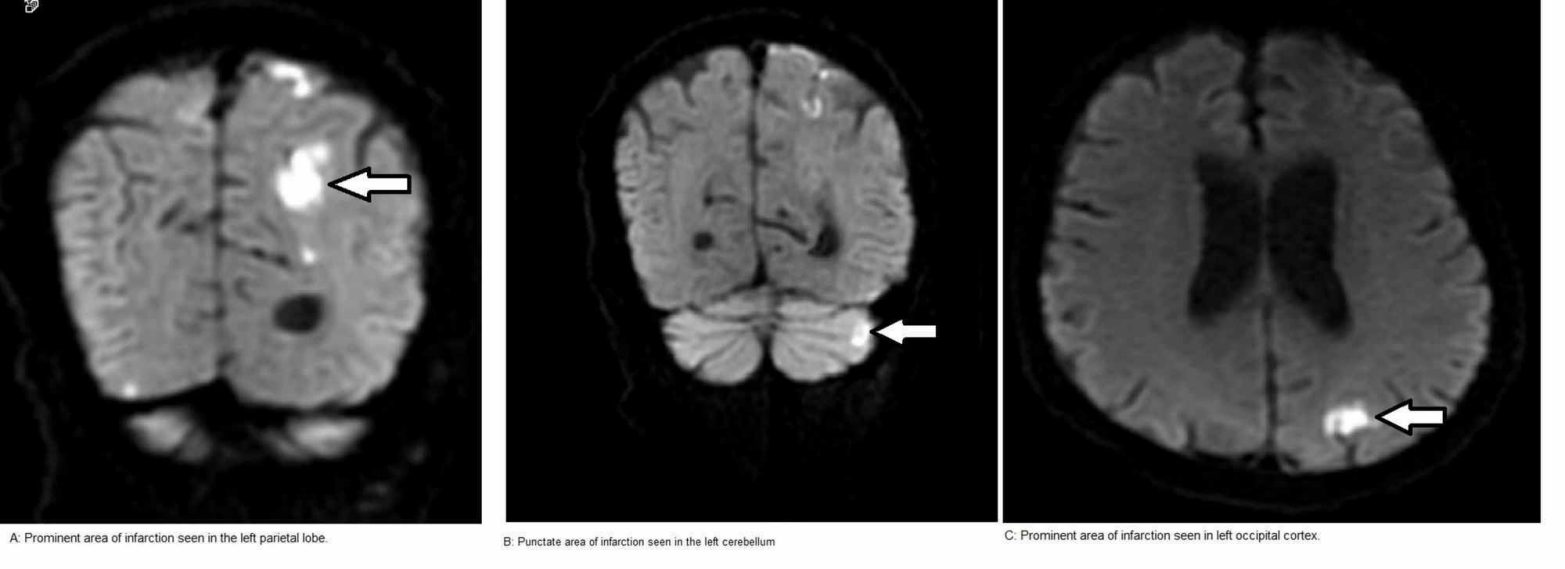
MRI of the head. Arrows showing multiple areas of infarct in left parietal (A) and occipital (C) cortex and left cerebellum (B).
